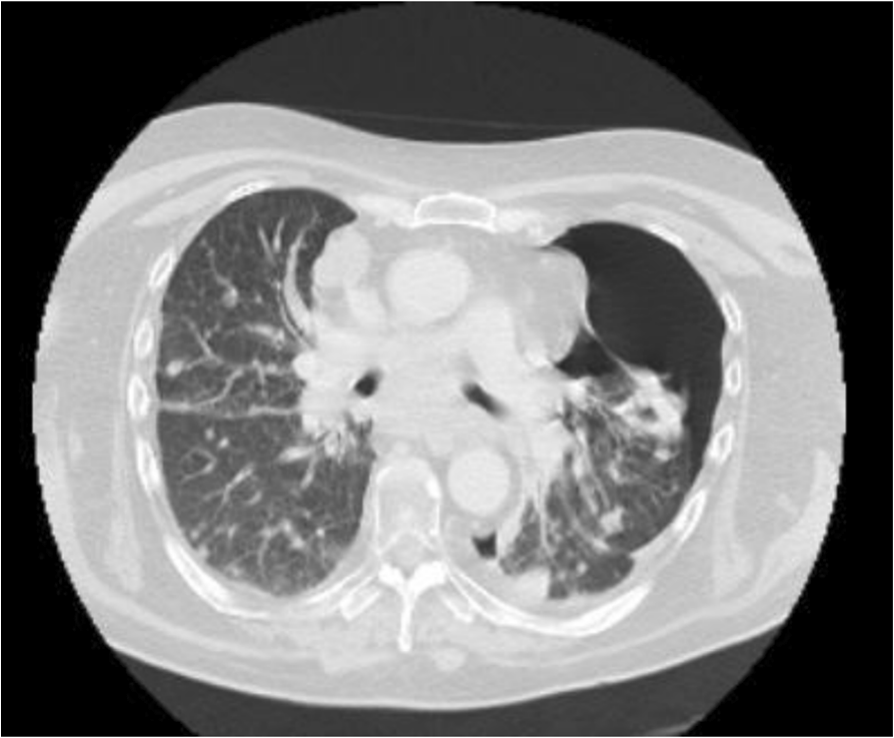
Chest CT showing disease response and a 50% left-sided pneumothorax. Staging CT scan following 4 weeks of treatment showing disease response and a 50% left-sided pneumothorax on ‘lung windows’.
